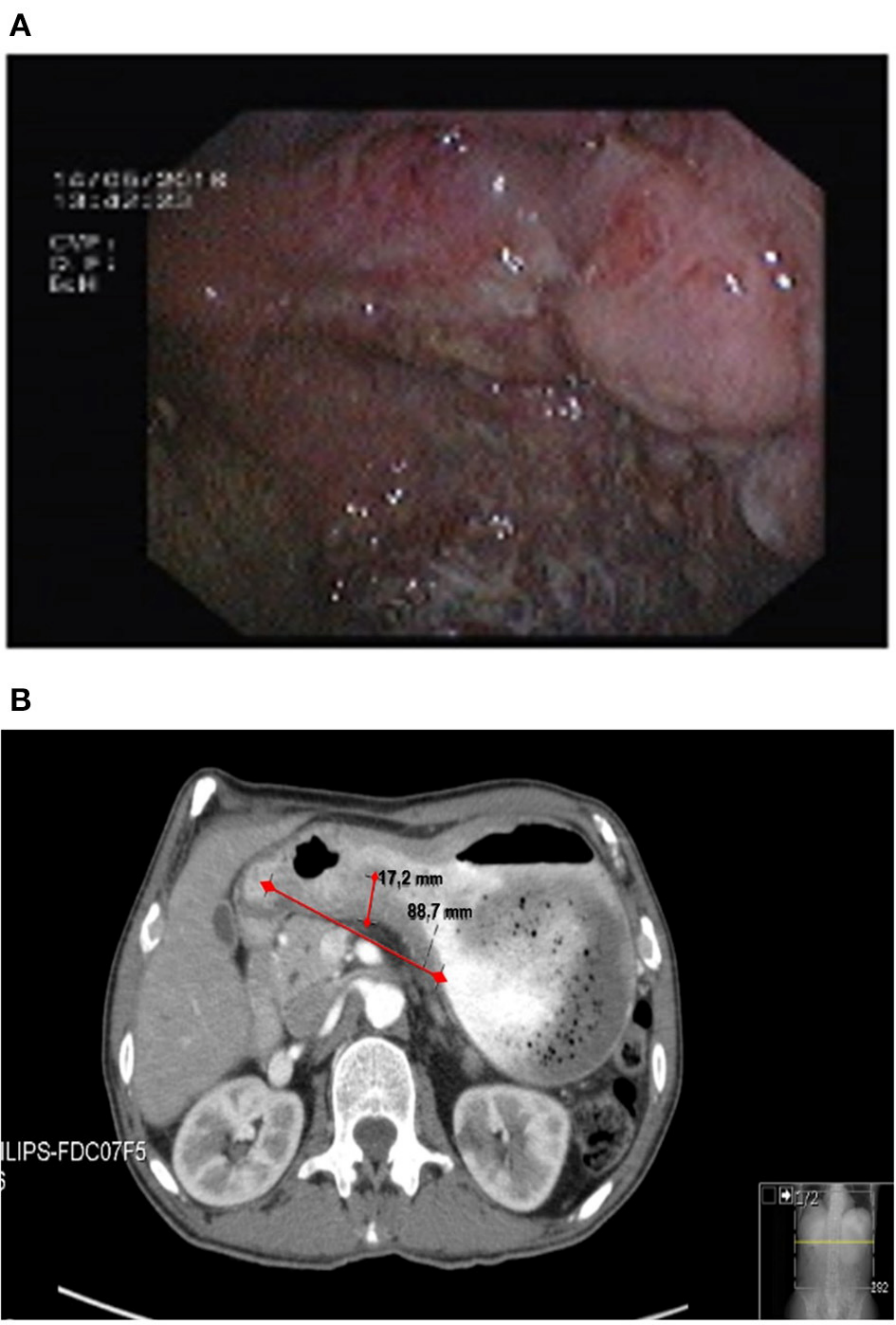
Gastric mass on (A) upper gastrointestinal endoscopy and (B) abdomen computed tomography (red arrow).
